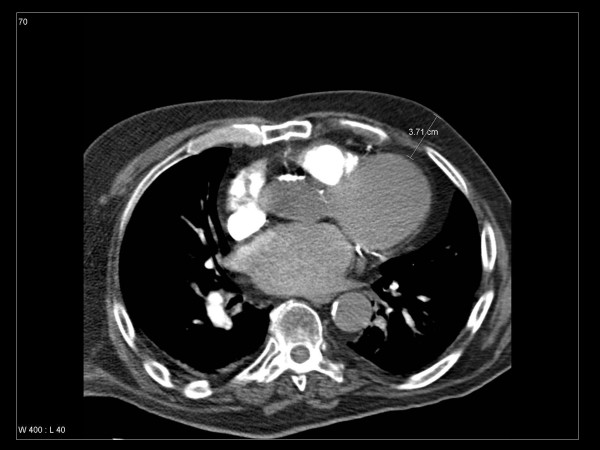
Axial contrast-enhanced computed tomography (CT) scan 3 cm caudal from the mamilla displaying the approximate depth of the 50% isodose from the CT-determined 9 MeV electron beam. In this 65-years-old non-cancer patient with previous heart disease, parts of the left ventricle would be exposed to unexpected doses of ionizing radiation.
